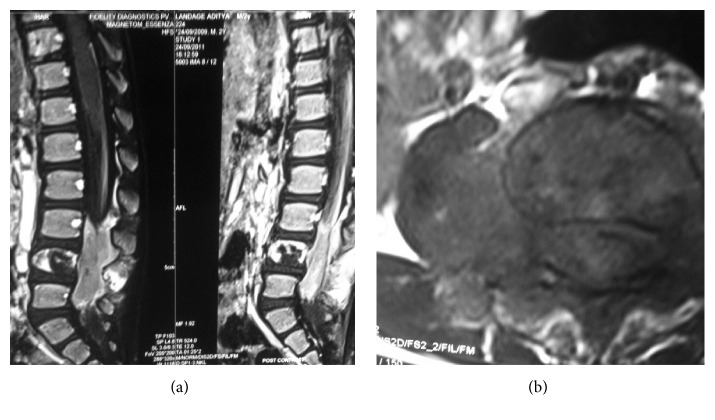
Sagittal projection of postcontrast T1 and T2 images demonstrating destruction of L4 vertebral body and tumour mass invading the spinal canal and posterior elements at L3, L4, and L5 vertebral levels. Axial projection of postcontrast T2 MRI image demonstrating tumour mass arising from the pedicle of L4 vertebra invading the spinal canal and the Right Psoas muscle.
